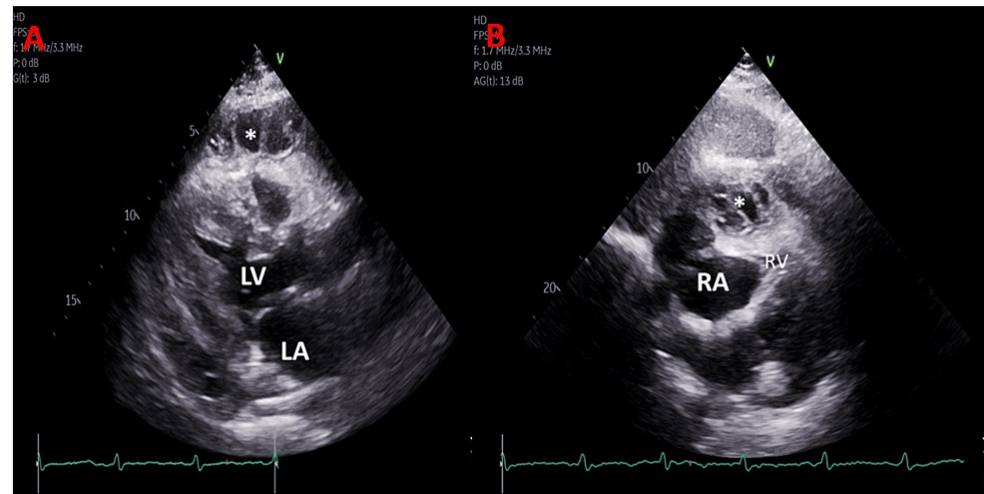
Transthoracic echocardiography shows pericardial effusion with multiple loculations (A) Parasternal long-axis view (B) Subcostal view. *pericardial effusion, LA: left atrium, LV: left ventricle, RA: right atrium, RV: right ventricle
